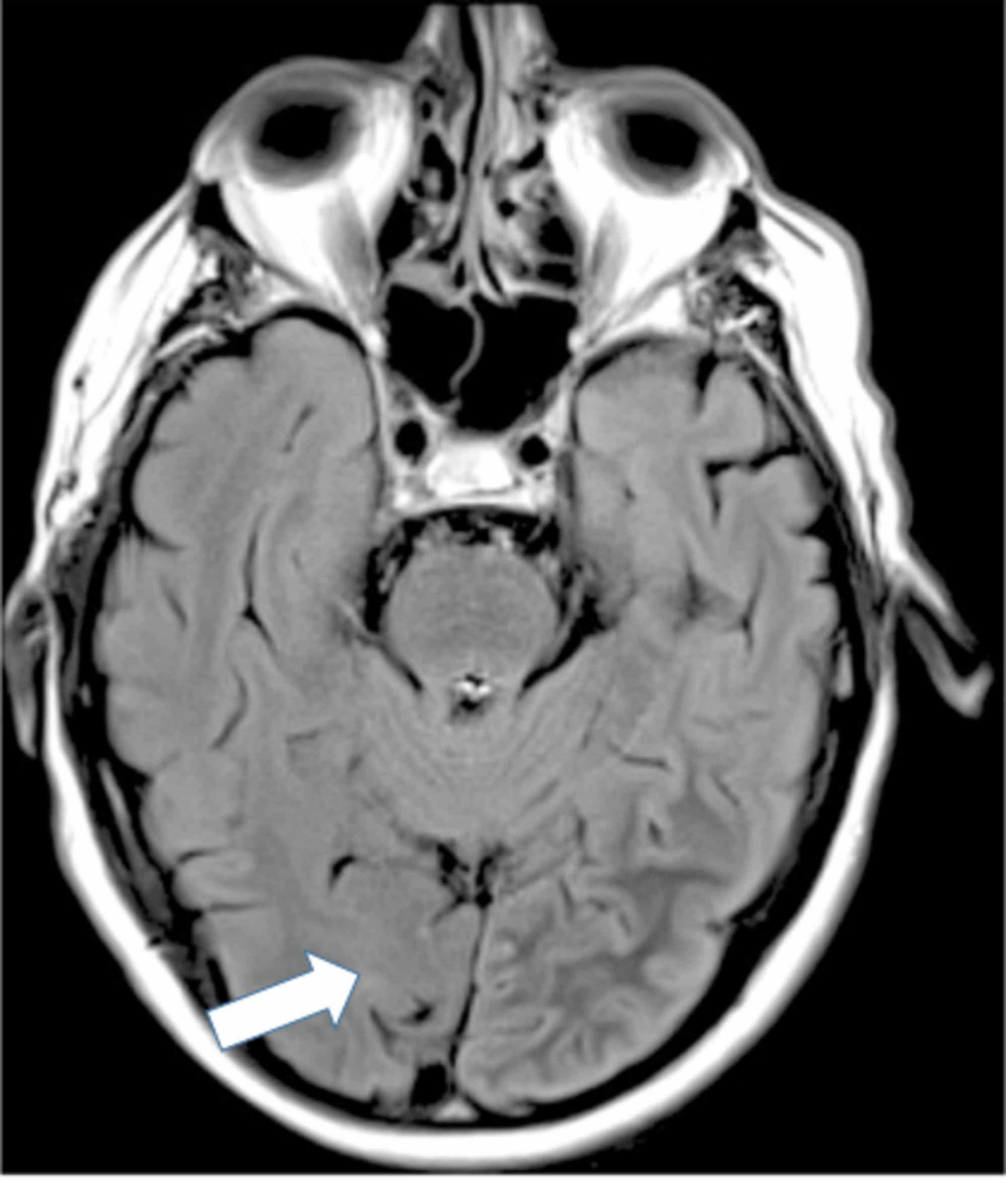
MRI of the brain demonstrating left parieto-occipital subcortical T2 hypointensity on fluid-attenuated inversion recovery in a patient with NKH-related occipital lobe seizure Courtesy: Dr. Swapna L, et al. MRI: magnetic resonance imaging; NKH: non-ketotic hyperglycemia
